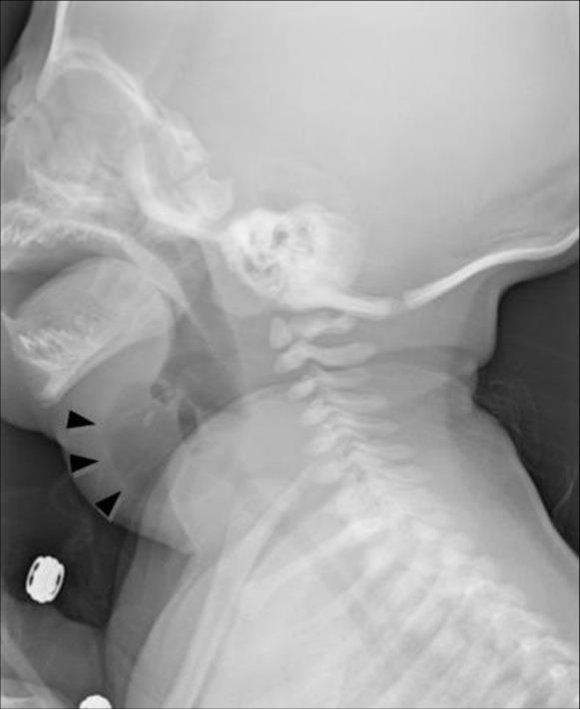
Figure 1: Left lateral neck X-ray finding : An air-fluid level within the mass in the left anterior neck. (Arrows)
